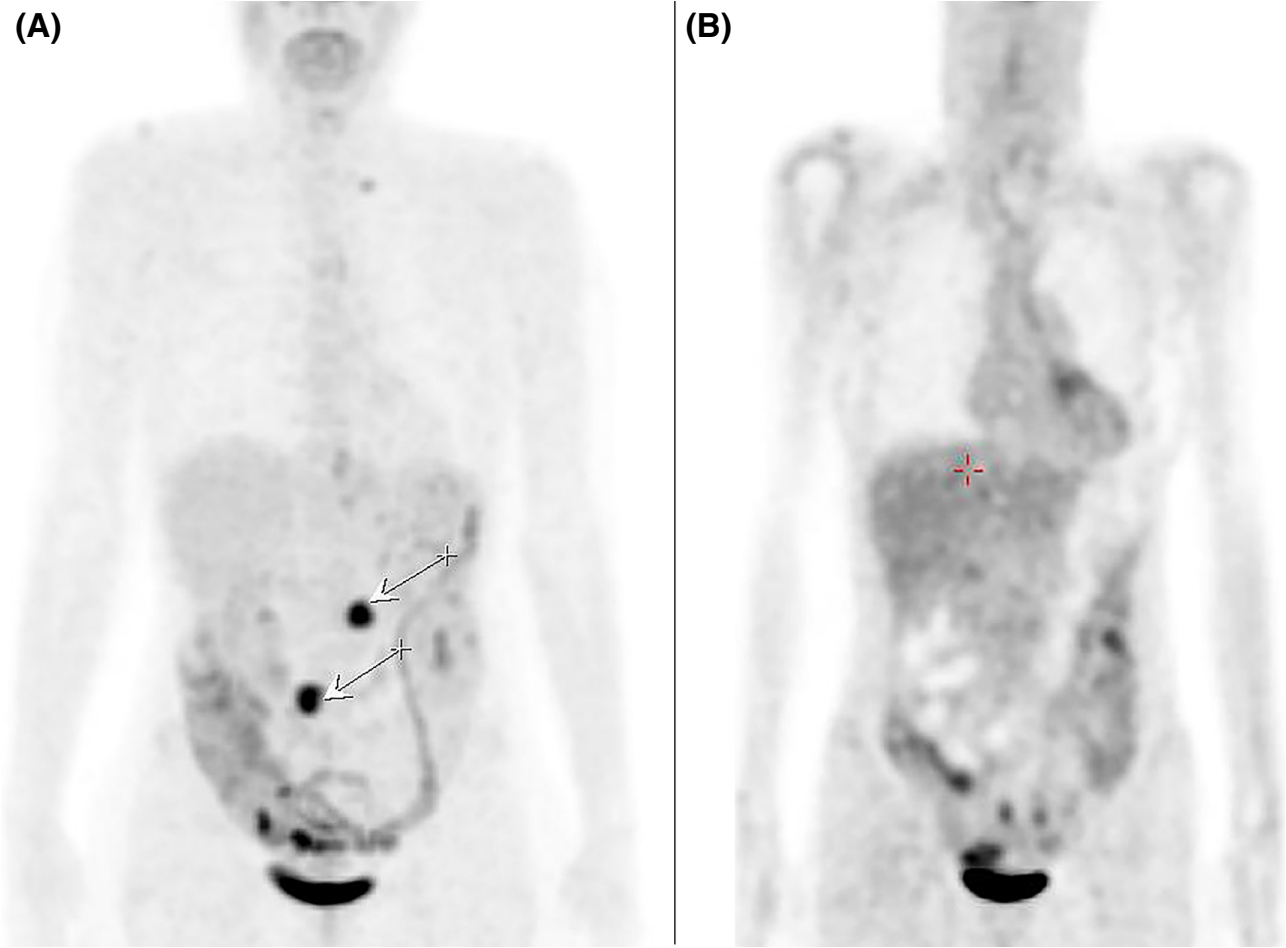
Images show the results of the fluorodeoxyglucose positron emission tomography/computated tomography (FDG‐PET/CT). A. discloses progressive disease with suspicious FDG‐uptake in two lymph nodes (arrows) pre IRECT treatment. B. shows the patient 6 weeks after IRECT treatment.
